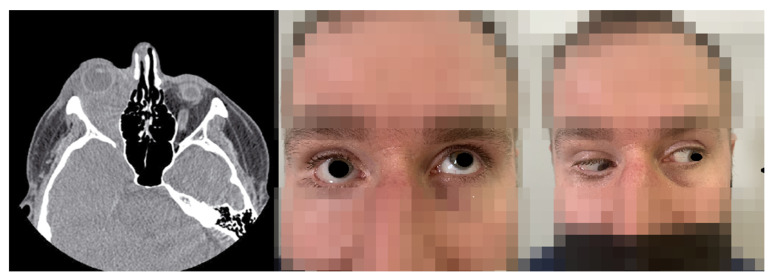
CT of the head and orbit. The image revealed a retrobulbar massive lesion on the right side. Proptosis of the right eye and RE motility restriction.
